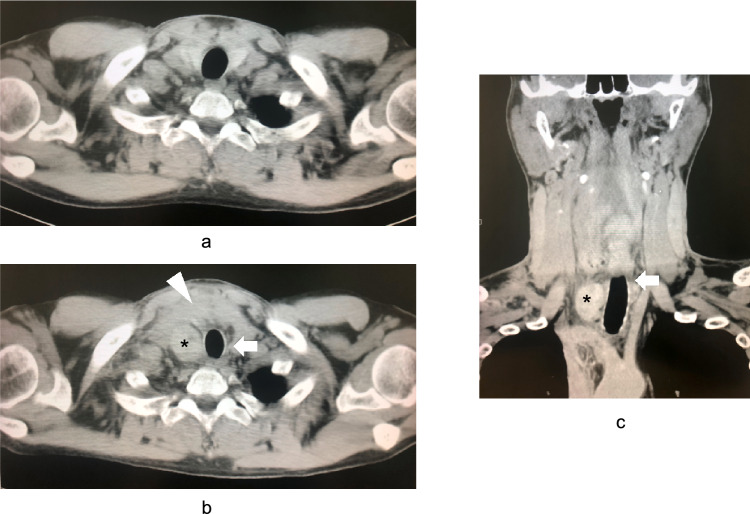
Computed tomography (CT) scan (a, b: axial section, c: coronal section). a: CT scan of the cervical region performed 9 days before the onset of cervical swelling showed no abnormal findings. b, c: Hematoma formation can be observed around the thyroid gland and in the upper mediastinum (white arrowhead), with a leftward shift and compression of the trachea (white arrow). A clearly defined high-density mass shadow can be observed on the right dorsal surface of the thyroid gland (black asterisk). Figure b and c were reproduced from Reference [7]
